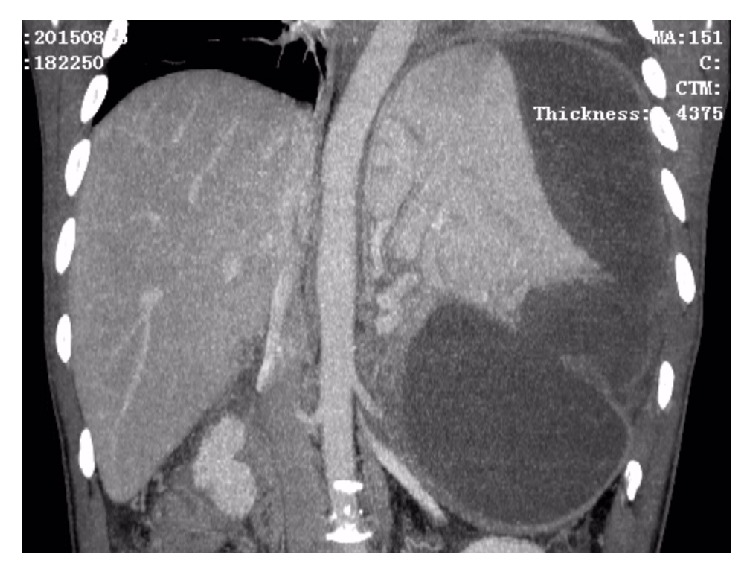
CT scan showing a large subcapsular splenic hematoma with a large pancreatic pseudocyst.
